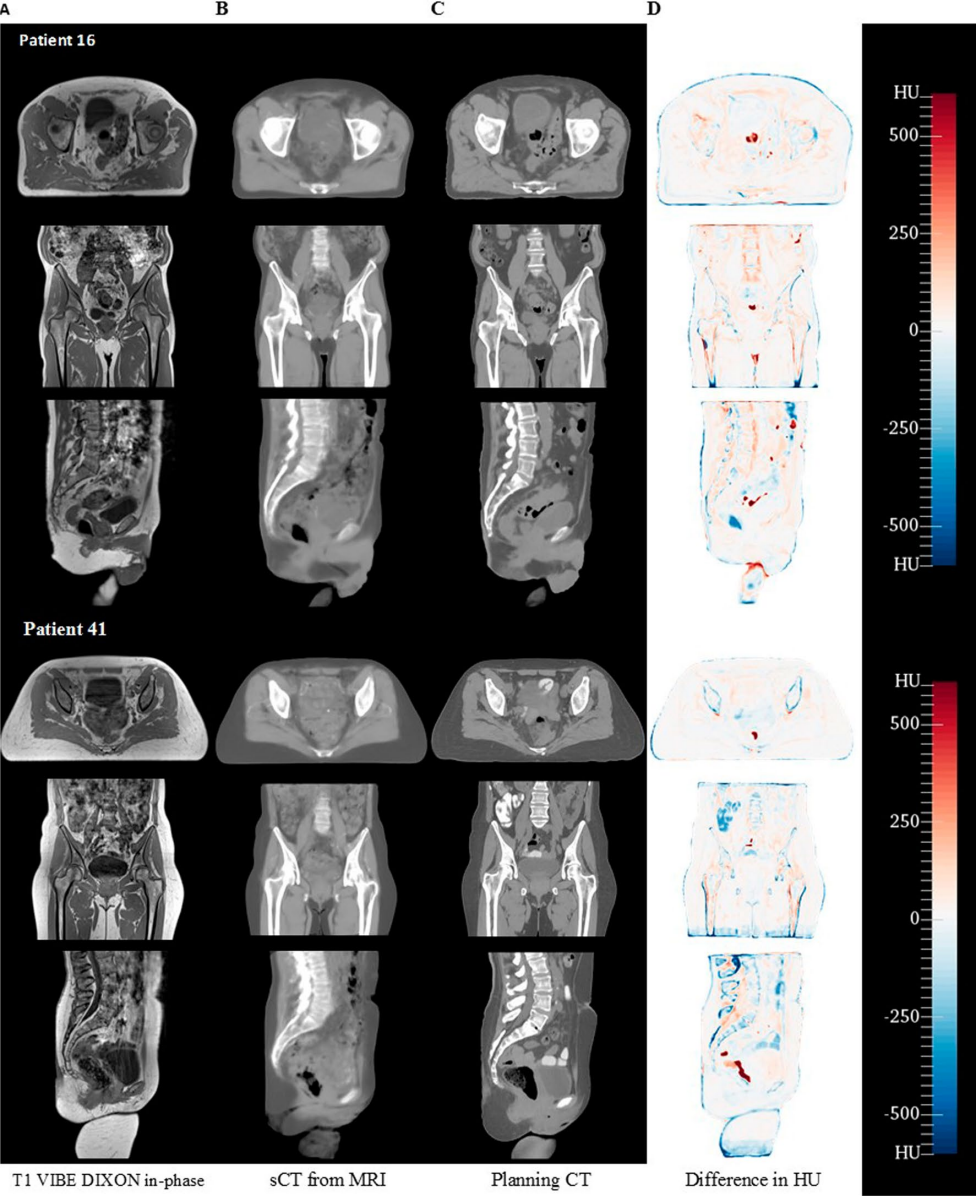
HU difference results for the best and worst performing gamma. Column A Original T1 VIBE DIXON in-phase MRI from patient 16 (best gamma) and patient 41 (worst gamma); B sCT generated from MRI; C planning CT scan; D HU difference between sCT and CT
